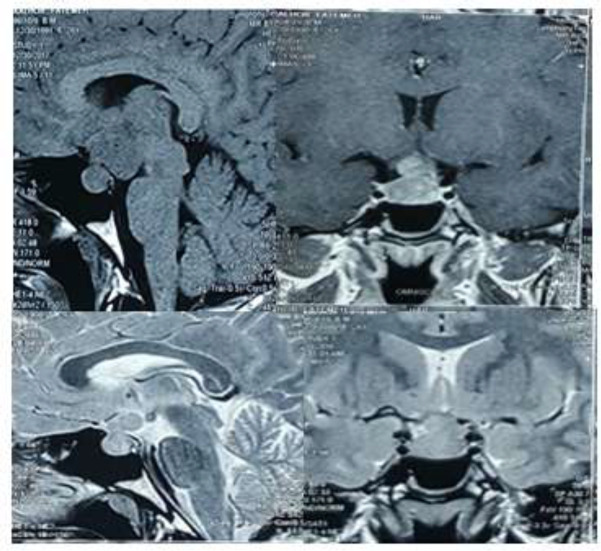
Multilobar sellar mass with heterogeneous enhancement and suprasellar growth, T1- and T2-weighted gadolinium-enhanced sagittal and coronal images
